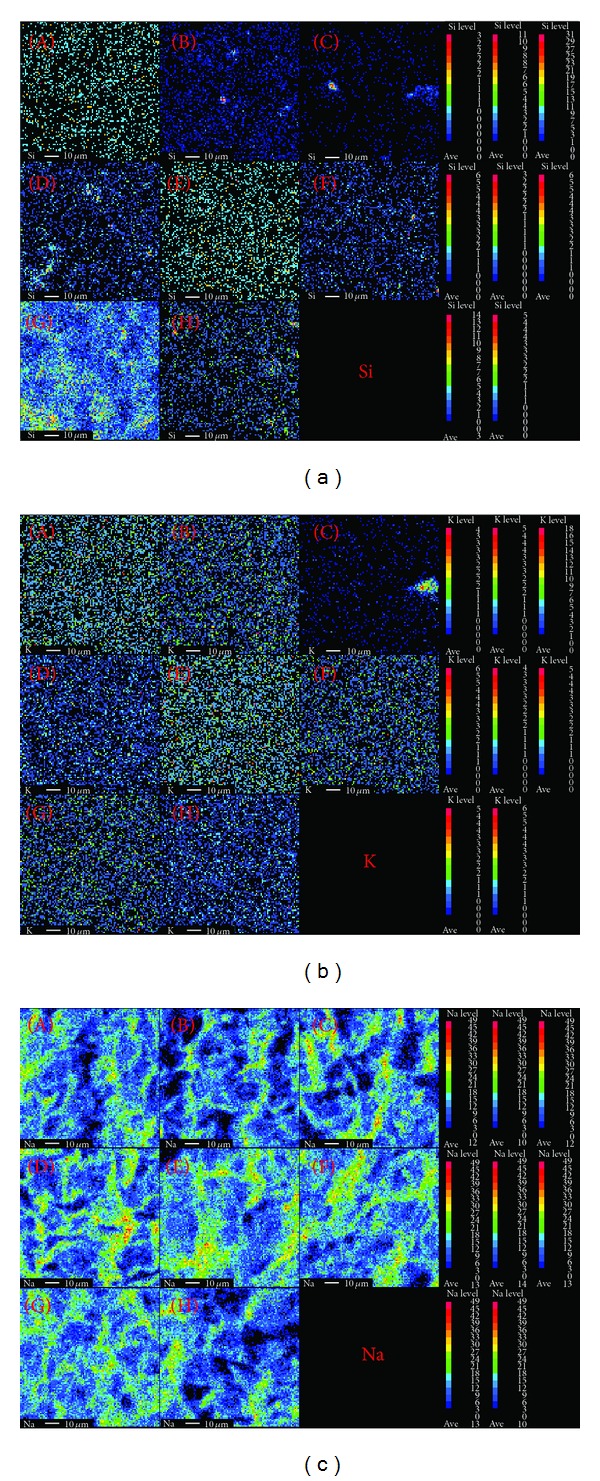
Wavelength dispersive X-ray analysis of Si, K, and Na in leaves of *A. multiflora* treated with silicon and NaCl. (A) MS medium, (B) MS + 1.8 mM Si, (C) MS + 3.6 mM Si, (D) MS + 7.2 mM Si, (E) MS + 50 mM NaCl, (F) MS + 50 mM NaCl + 1.8 mM Si, (G) MS + 50 mM NaCl + 3.6 mM Si, and (H) MS + 50 mM NaCl + 7.2 mM Si.
